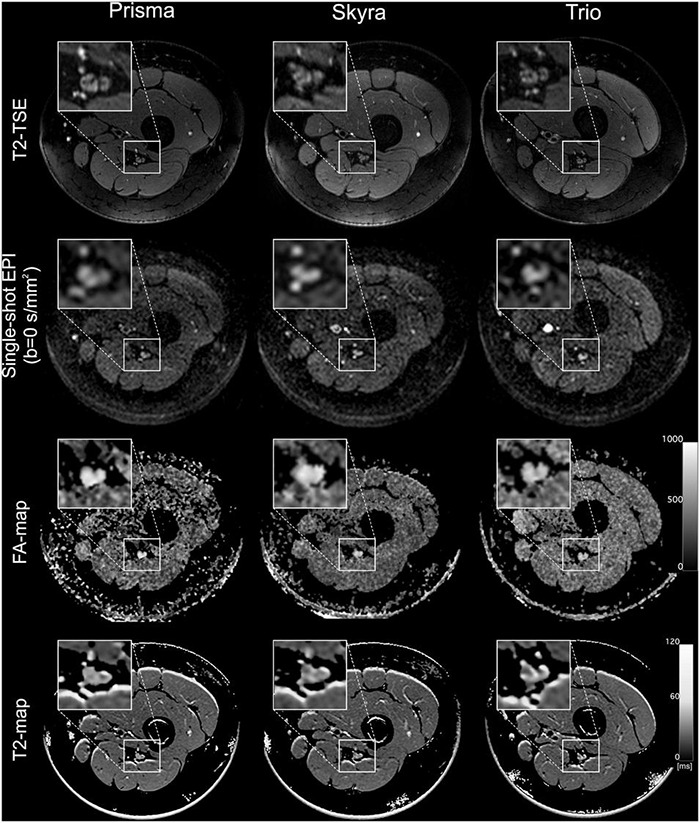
MR imaging of the left leg at mid-thigh level acquired in the same individual on three different MR scanners (Prisma, Skyra, and Trio). From top to bottom, the rows show representative images for a T2 turbo spin echo (TSE) sequence, a single-shot echo planar imaging sequence (b0-image), a calculated FA-map and an exemplary T2-map generated by the OsiriX plugin T2 map. Insets show a magnification of the sciatic nerve.
